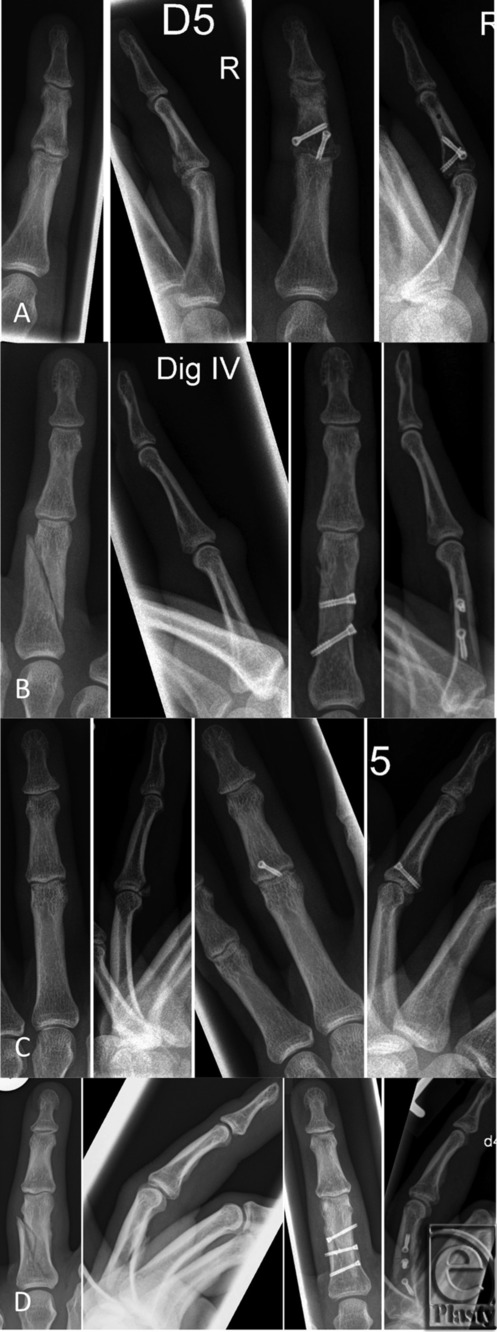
Four different cases (row a-d) with pre- and postoperative radiographs. (a) Intra-articular PIP joint fracture of the middle phalanx with 2 screws. (b) Typical extra-articular displaced oblique fracture treated with 2 screws. (c) Multiple fragments in the proximal phalanx treated with 3 screws, which is certainly a limitation for this type of osteosynthesis. (d) Mulifragment proximal phalanx fracture treated with 3 screws.
